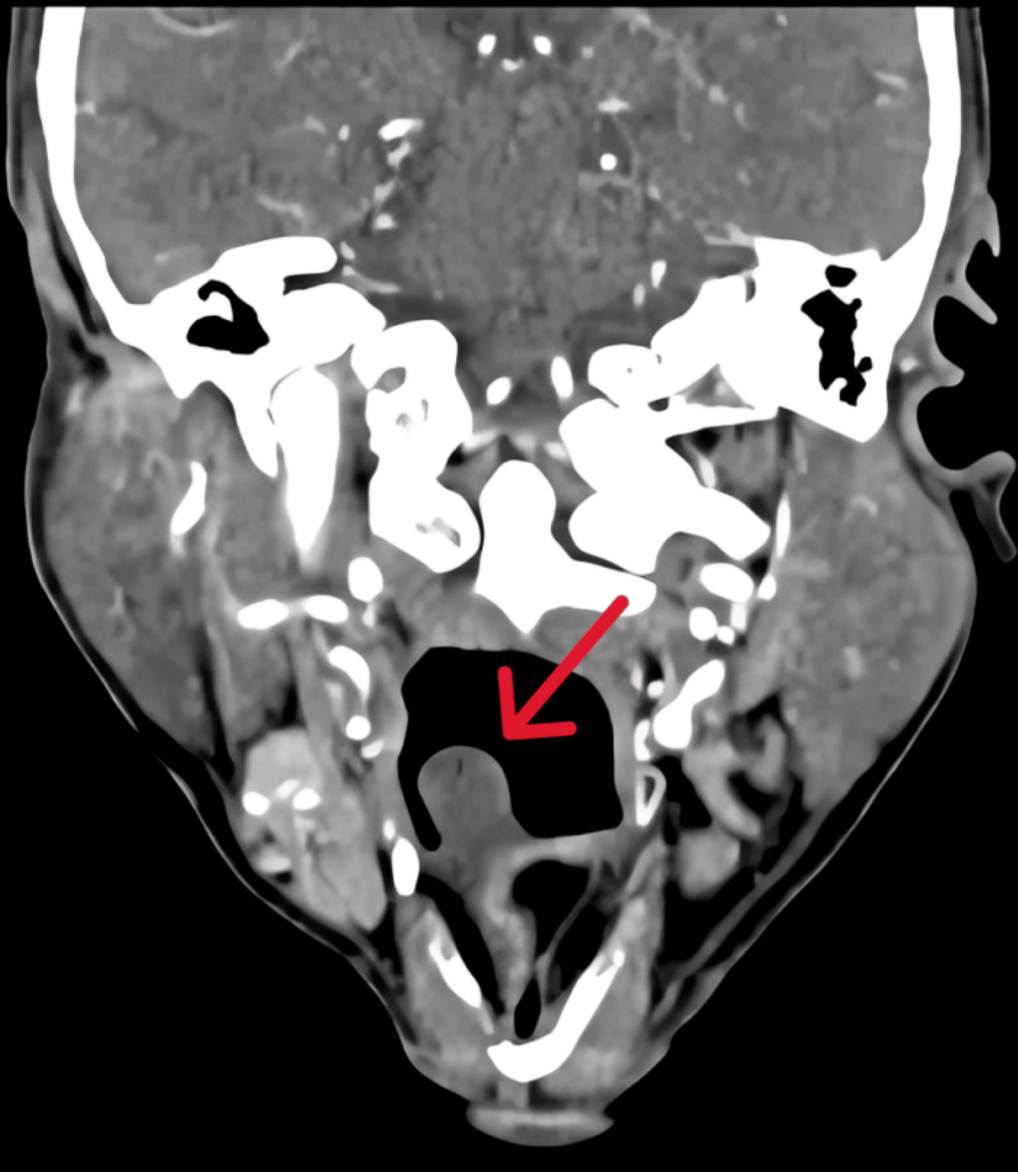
Computed tomography of the neck, where the coronal cuts show the cyst arising from vallecula (red arrow)
